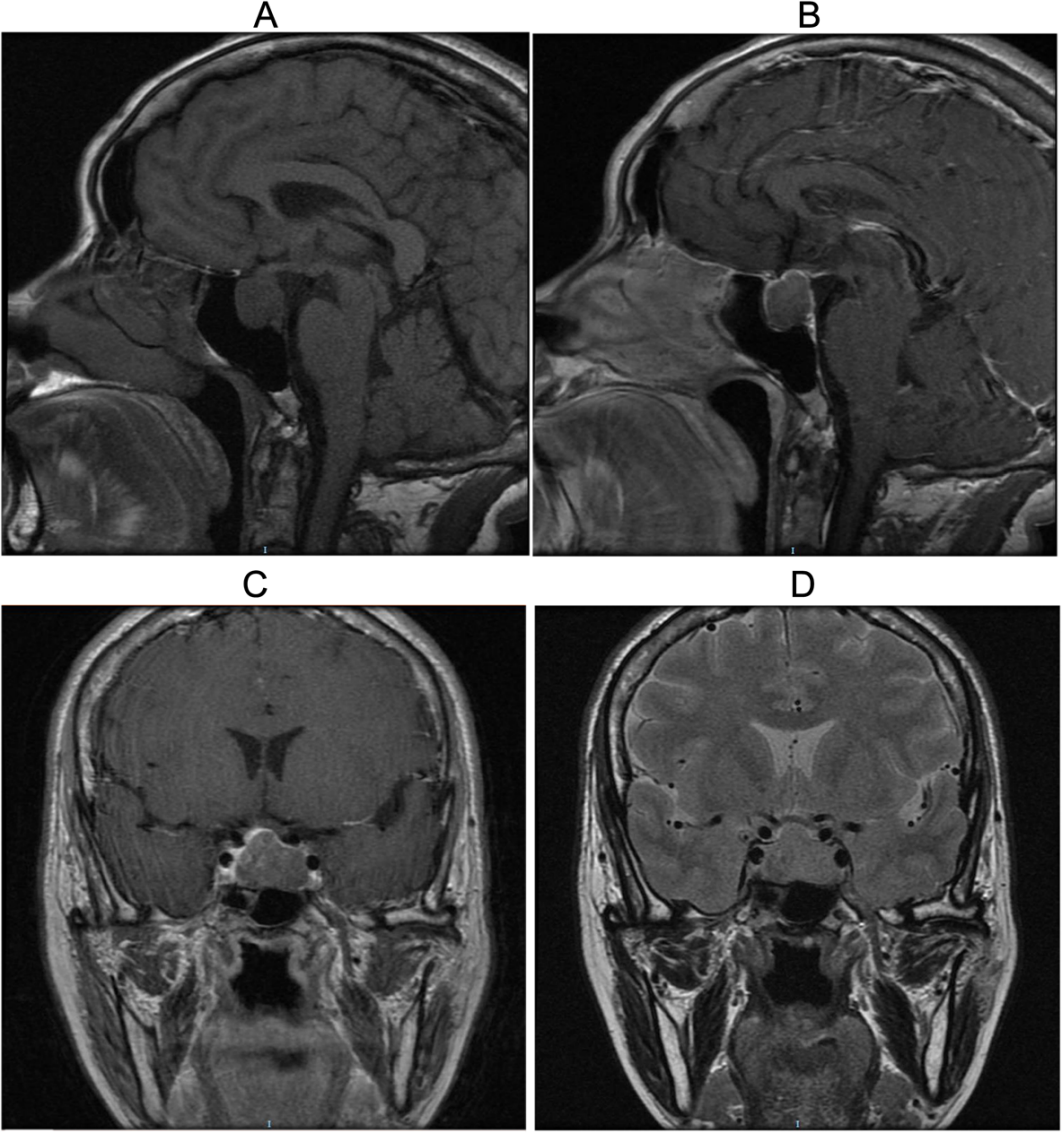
Representative image of a pituitary adenoma detected in magnetic resonance imaging. The sella is expanded within which a pituitary adenoma is visible. T1-weighted images showed uniform signals (a) and were slightly enhanced with contrast (b and c), whereas T2-weighted images had slightly higher signals (d)
